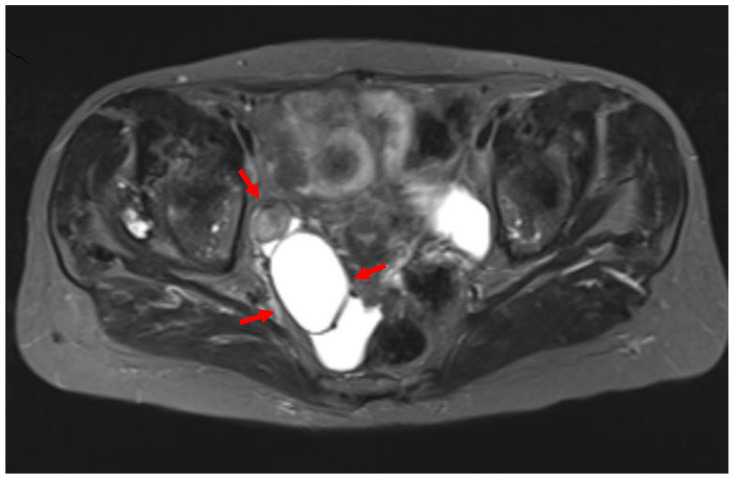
Magnetic resonance imaging of primary carcinoma of the fallopian tube (red arrows), which both preoperatively and intraoperatively was misdiagnosed as a benign ovarian tumor (our case).
